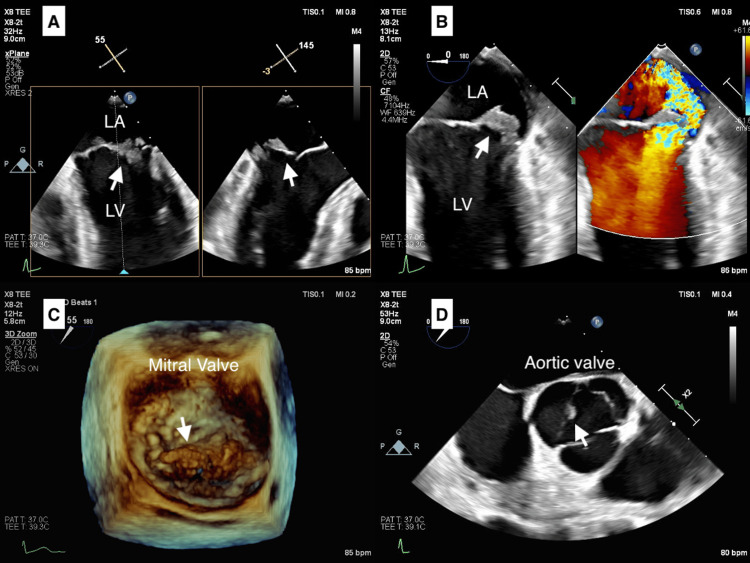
Transesophageal echocardiogram (TEE) (A) TEE shows vegetation on the mitral valve. (B) Severe mitral regurgitation. (C) 3D view of vegetation on the mitral valve. (D) Vegetation on the non-coronary cusp of the aortic valve.
